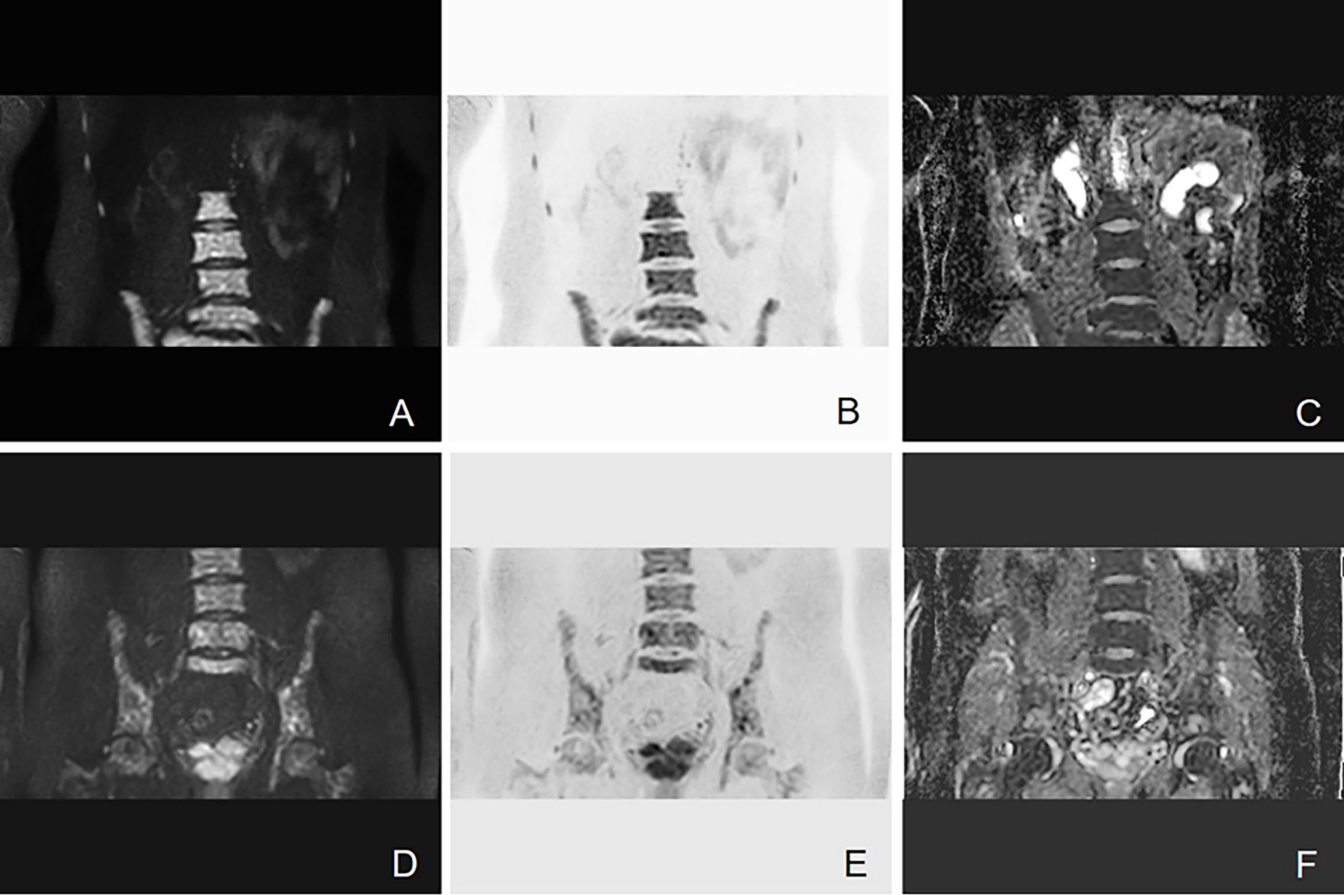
Example of DW-MRI image with treatment response of SD. A case of a 52-year-old man who was diagnosed with MM (IgA kappa light chain, RISS Stage III) is presented here. (A–C) Coronal images of DWI, inverted images, and ADC map at baseline visit. (D–F) Corresponding images of the same patient after four courses of induction chemotherapy (three courses of bortezomib, cyclophosphamide, and dexamethasone + one course of bortezomib, ralidomide, and dexamethasone). The efficacy of induction chemotherapy was evaluated as SD. Diffuse abnormal signal can be seen in the lumbar spine and pelvis. It has been shown that the high signal in the bone marrow of DWI sequence slightly decreased after induction chemotherapy. The mean ADC value of lesions in the scan range also increased from 0.693 × 10^−3^ to 0.775 × 10^−3^ mm^2^/s.
